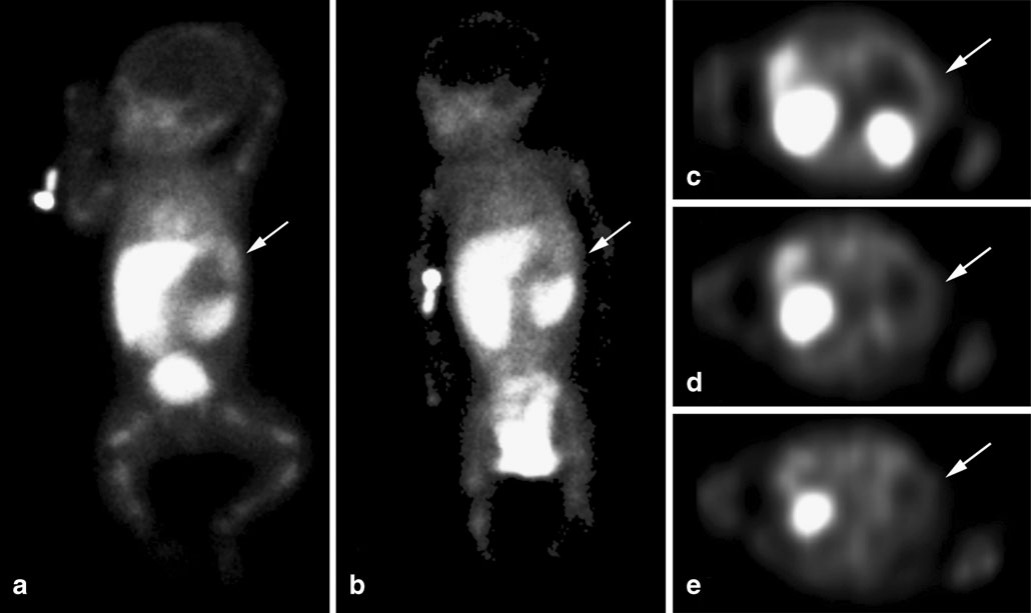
Antigranulocyte antibody-scintigraphy with 100 MBq (99 m-)Tc-sulesomab shows a photopenic area in the left upper quadrant of the abdomen surrounded by a rim of increased radioactivity uptake (*arrows*) and physiological accumulation of radioactivity in the myocardium, liver, spleen, kidneys, bladder and bone marrow. a Static image (anterior view) 1 h postinjection. b Static image (anterior view) 6 h postinjection. c–e SPECT-images 6 h postinjection
